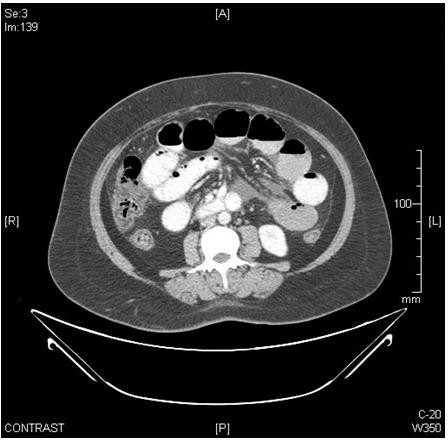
Axial contrast-enhanced computed tomography image shows dilated bowel loops.
